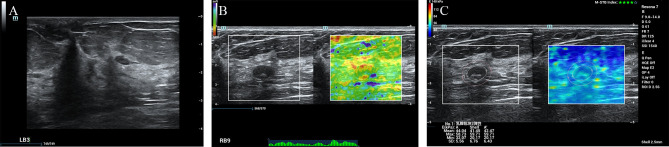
The fibroadenoma was downgraded by the ES of 1-3 points and the Emax-A’-S2.5 below 80kpa. A 67-year-old female patient had a lesion on her right breast. Pathologically confirmed fibroadenoma. The size of the lesion was 11.9mm and ultrasound images of the breast lesion were evaluated as BI-RADS 4a (A), with an elasticity score of 2 (B), Emax-A of 58.7kpa and Emax-A’-S2.5 of 59.71kpa (C).
